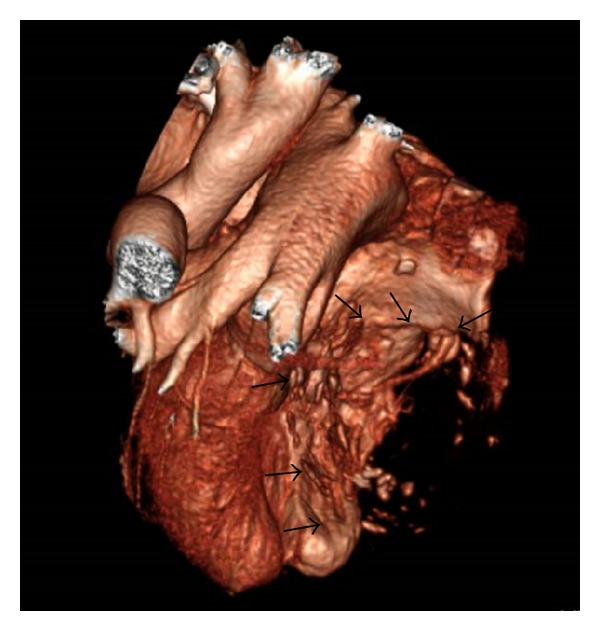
Volume rendering Computed Tomography (CT) image, obtained by the exclusion of the mass (arrows), better demonstrates the effect of the lesion on the heart, in particular the markedly impressed and dislocated cardiac walls.
